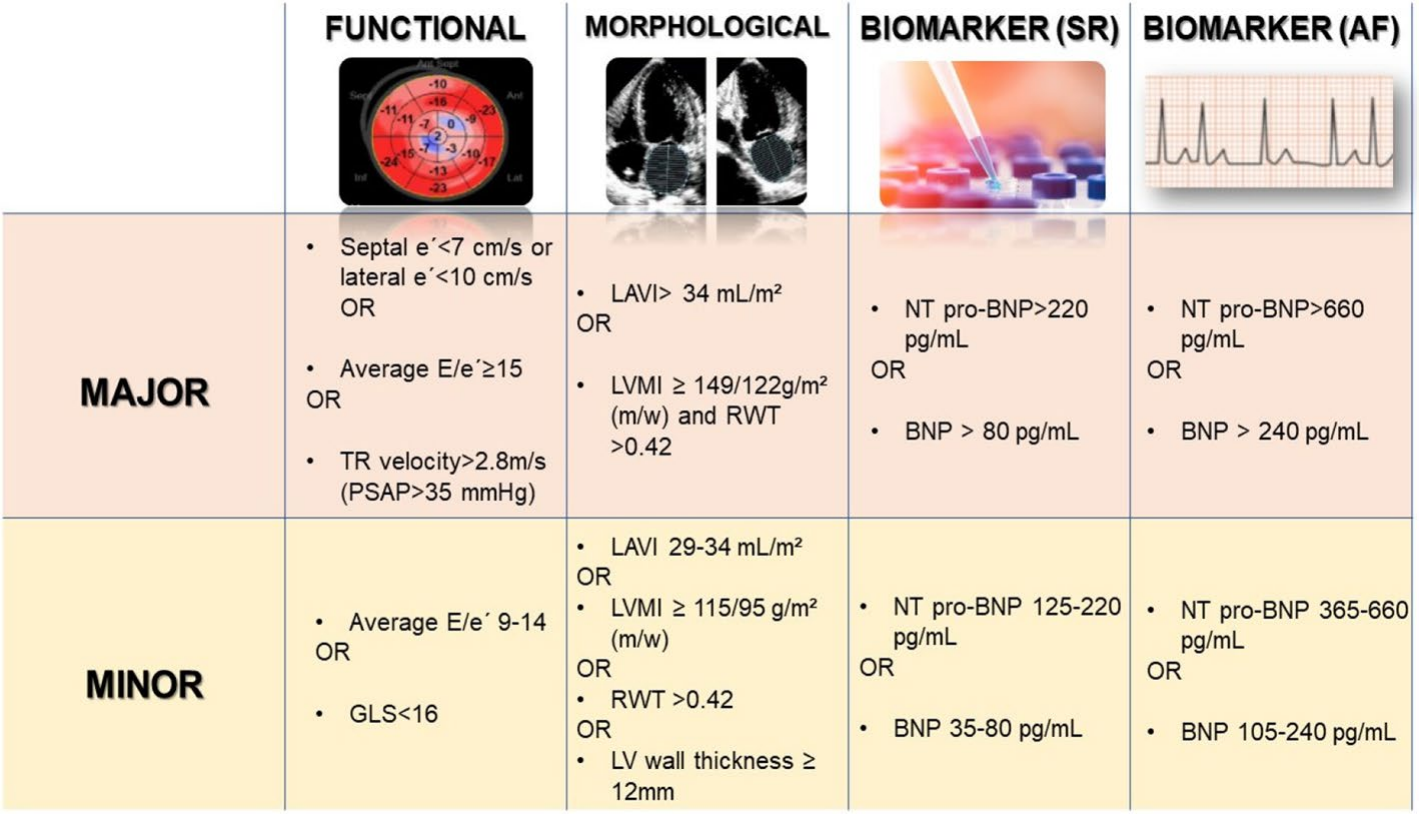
Echocardiographic and natriuretic peptide heart failure with preserved ejection fraction workup and scoring system. TR: Tricuspid Regurgitation. LAVI: Left Atrial Volume Index. LVMI: left ventricular mass index. RWT: Relative Wall Thickness. M: Men. W: Women. SR: Sinus Rhythm.AF: atrial fibrillation. Adapted from: Pieske, B et al. How to diagnose heart failure with preserved ejection fraction: the HFA–PEFF diagnostic algorithm: a consensus recommendation from the Heart Failure Association of the European Society of Cardiology
